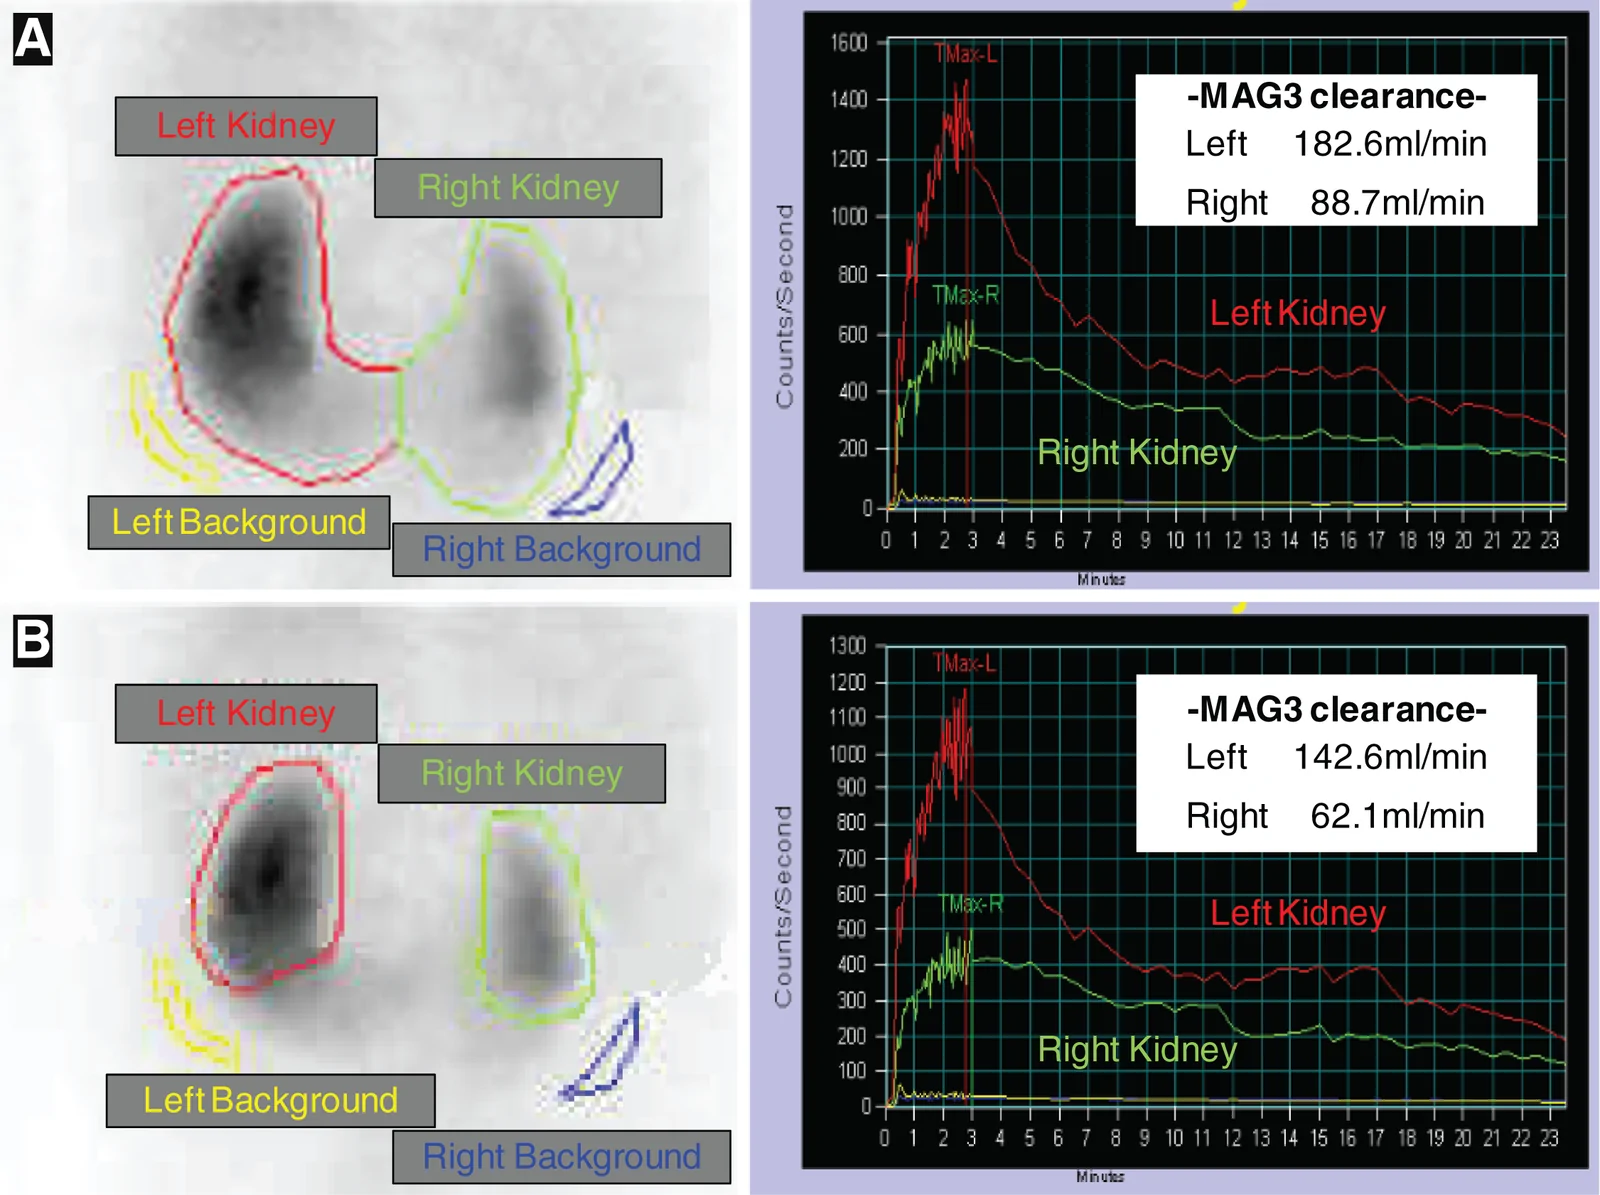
(A) Renal scintigraphy shows that bordering the isthmus of the horseshoe kidney, the MAG3 clearance of the left kidney was 182.6 mL/min, while that of the right kidney was 88.7 mL/min, indicating a left-sided predominance in renal function. (B) Excluding the isthmus of the horseshoe kidney, the MAG3 clearance was 142.6 mL/min for the left kidney and 62.1 mL/min for the right kidney. These results indicate that the MAG3 clearance of the isthmus was 66.6 mL/min. MAG3, mercaptoacetyltriglycine
